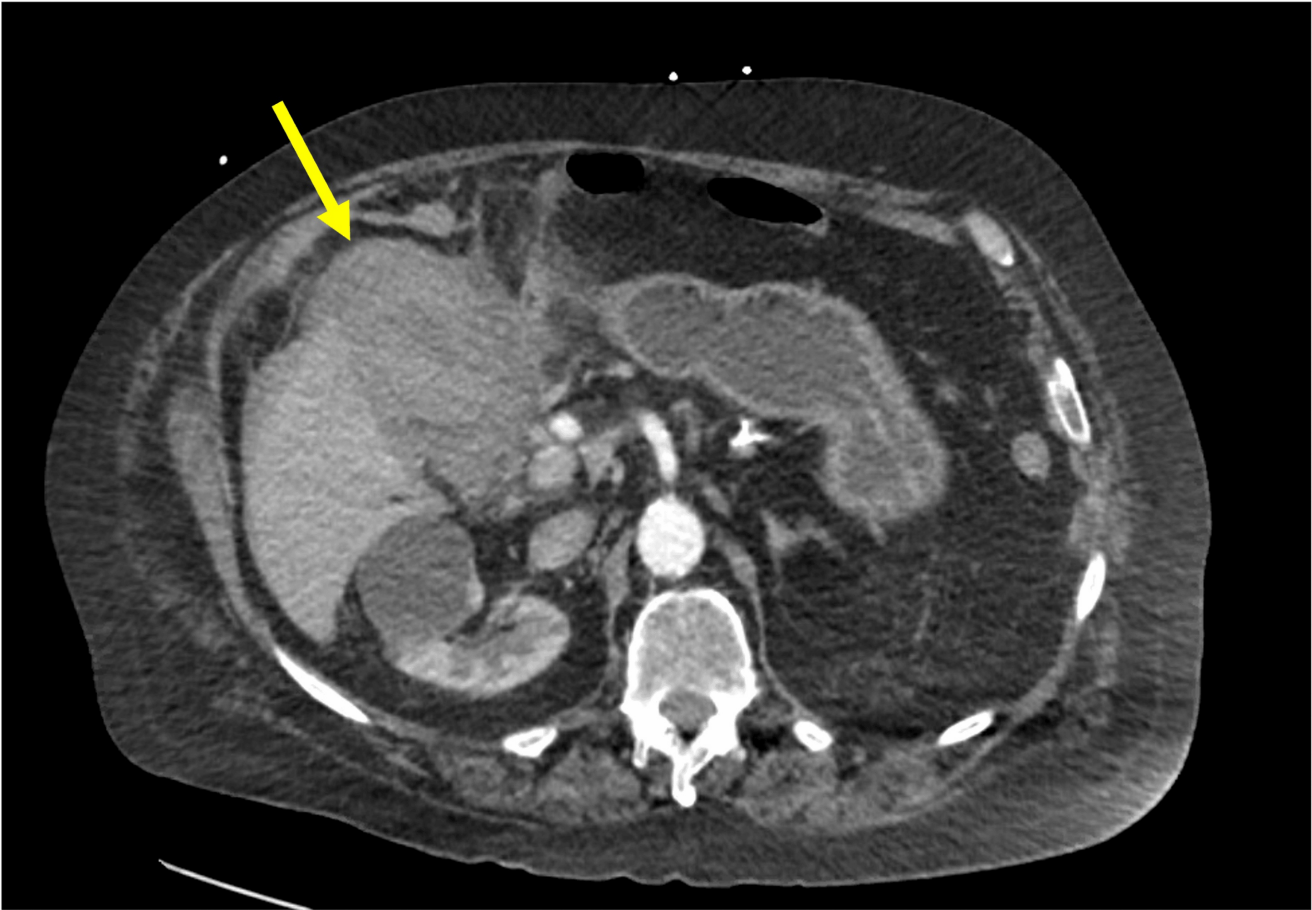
Axial abdominal CT scan showing gallbladder wall thickening and significant distention (yellow arrow).
